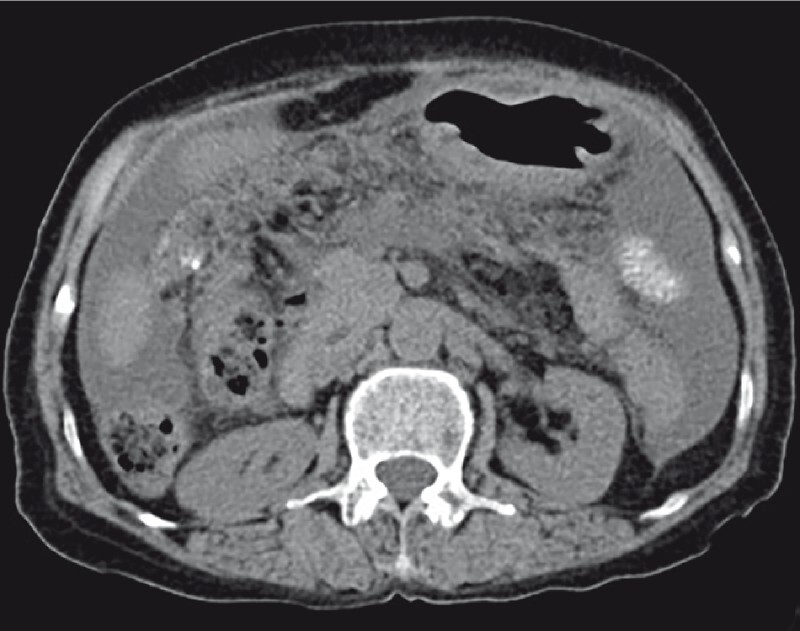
Computed tomography image showing an abdominal effusion, with blurring of the abdominal fat space.
